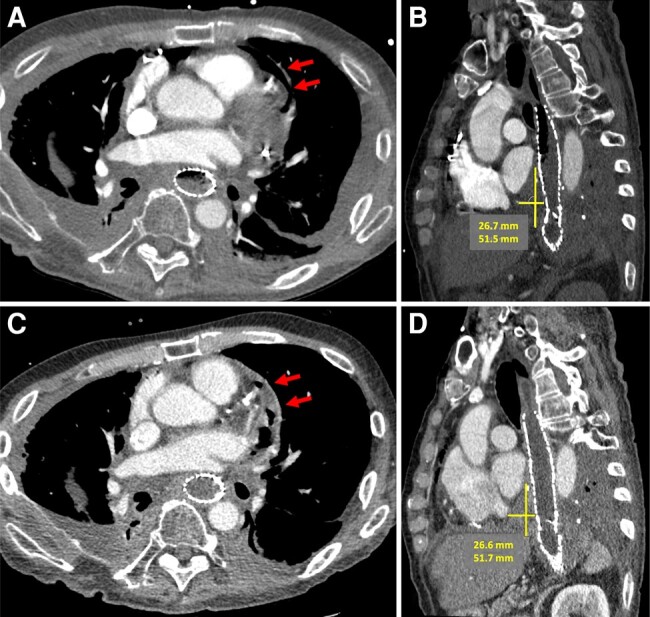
Serial CT imaging of mediastinal sarcoma and pericardium. (*A*) Axial view, CT angiogram of chest showing simple, homogenous pericardial effusion (arrows) and pneumopericardium with pericardial drain in place, 6 days after mediastinal RT. (*B*) Sagittal view, CT chest demonstrating a 27 × 52 mm posterior mediastinal sarcoma (margins demarcated by lines) exerting mass effect to the left atrium and oesophagus (status post oesophageal stent), 6 days after mediastinal RT. (*C*) Axial view, repeat CT with contrast showing increased, complex gaseous and heterogeneous pericardial effusion, pericardial thickening (arrows), and pneumopericardium, 21 days after mediastinal RT. (*D*) Sagittal view, repeat CT chest redemonstrating similar sized sarcoma and thickened pericardium, 21 days after mediastinal RT.
